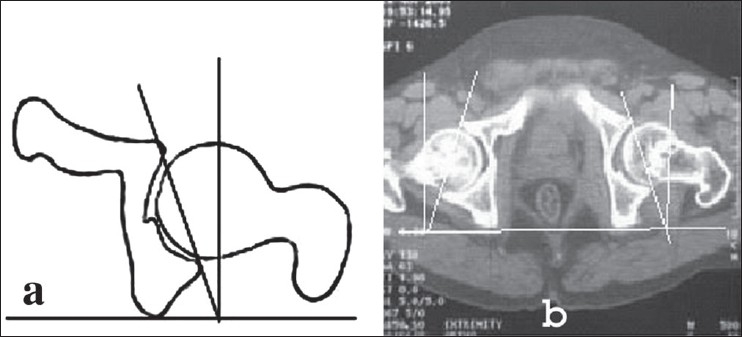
Line diagrm and (a) CT scan (b) of acetabular version showing increased acetabular version on left side -20 degree
